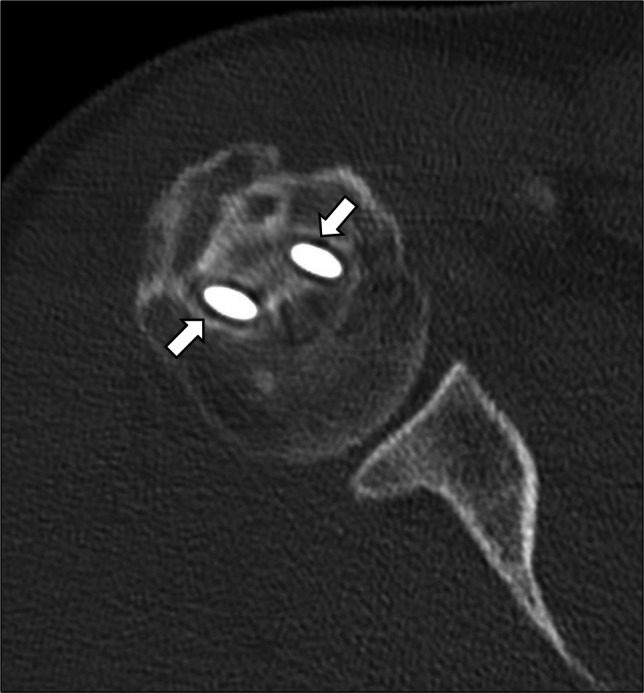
Hardware loosening in a 42-year-old man. After curettage of an ACT of the proximal humerus, bone grafting and internal fixation, CT showed a lucency exceeding 2 mm (arrows) around the screws at 2-year follow-up
